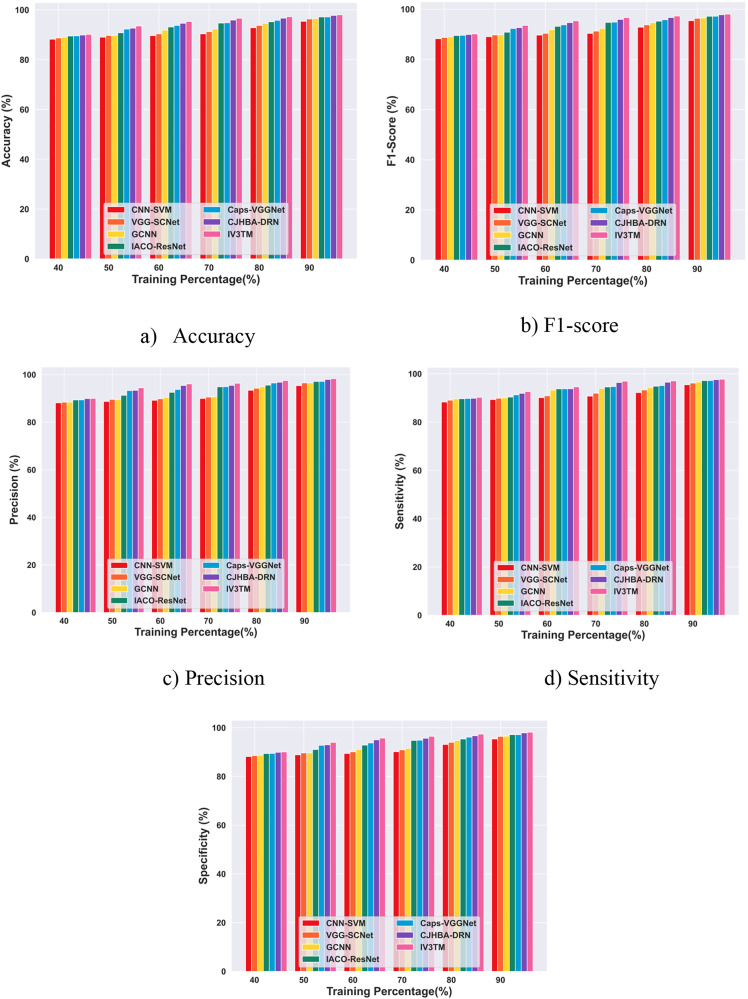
Comparative Evaluation with Training Percentage based on the Brain MRI dataset.
